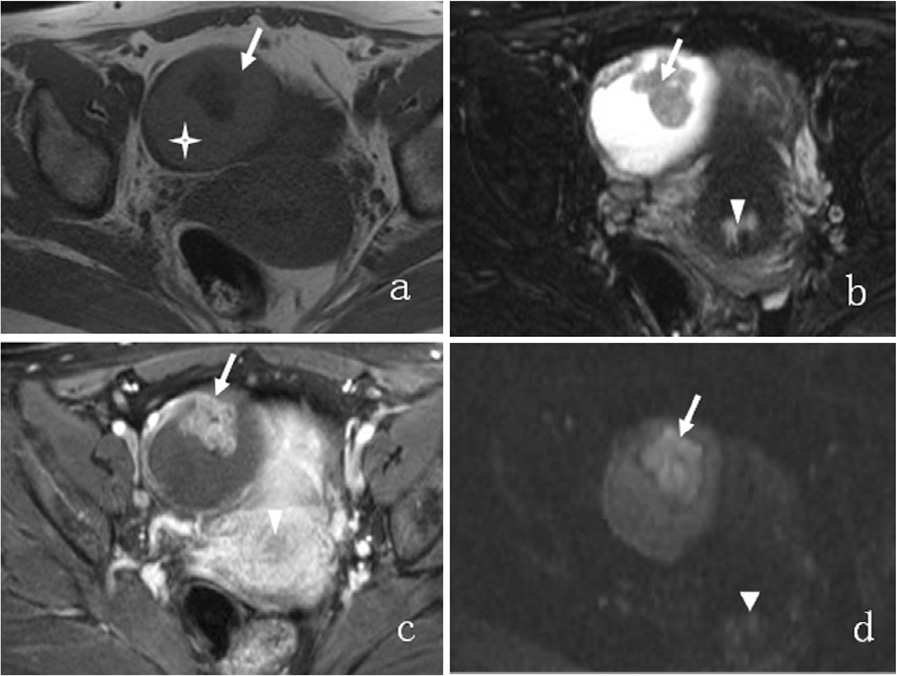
A 50-year-old woman with right-sided OEC. The tumor appears as a mainly cystic mass with a large mural nodule. The cystic component (crossstar) shows slight hyperintensity on axial T1WI (a), homogeneous hyperintensity on axial T2WI with fat suppression (b), no enhancement on contrast-enhanced T1WI with fat suppression (c), isointensity on DWI (d). The mural nodule (arrow) demonstrates isointensity (a), heterogenous hyperintensity (b), marked enhancement (c) and hyperintensity (d) with an ADC value of 1.030 × 10^−3^ mm^2^/s, respectively. Synchronous uterine endometrial carcinoma (arrowhead) shows mild enhancement on contrast-enhanced T1WI and hyperintensity on DWI.
